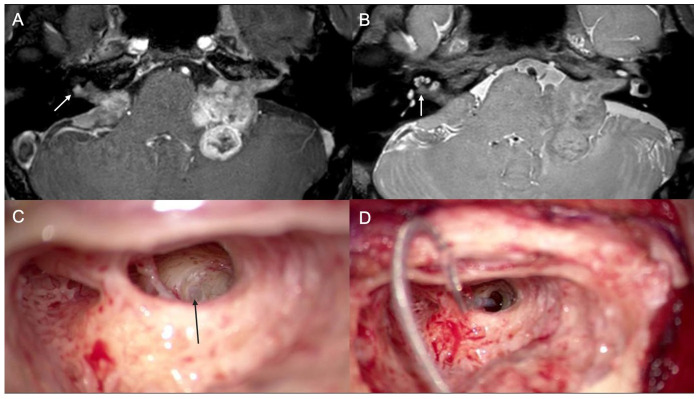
Case #1. Preoperative T1-weighted MR with gadolinium enhancement (A) and proton density-weighted MR image (B) show a small mass in the basal turn of the cochlea (white arrows). Through the round window, a soft tissue mass is shown (black arrow) (C). After removal of the mass, the electrode of the cochlear implant is introduced and fully inserted (D).
